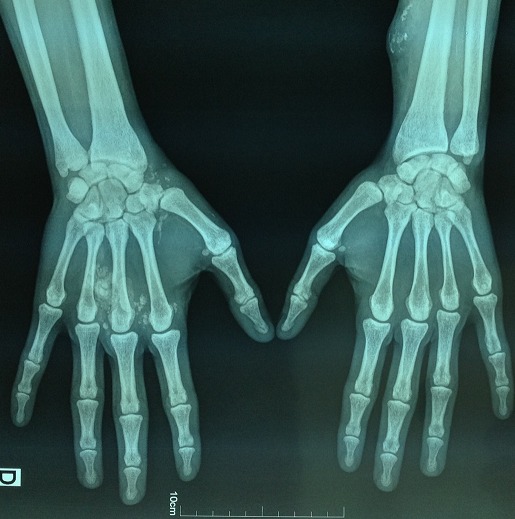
Radiographie standard objectivant la régression de la calcinose pseudo tumorale de la main droite quatre mois après para thyroïdectomie
